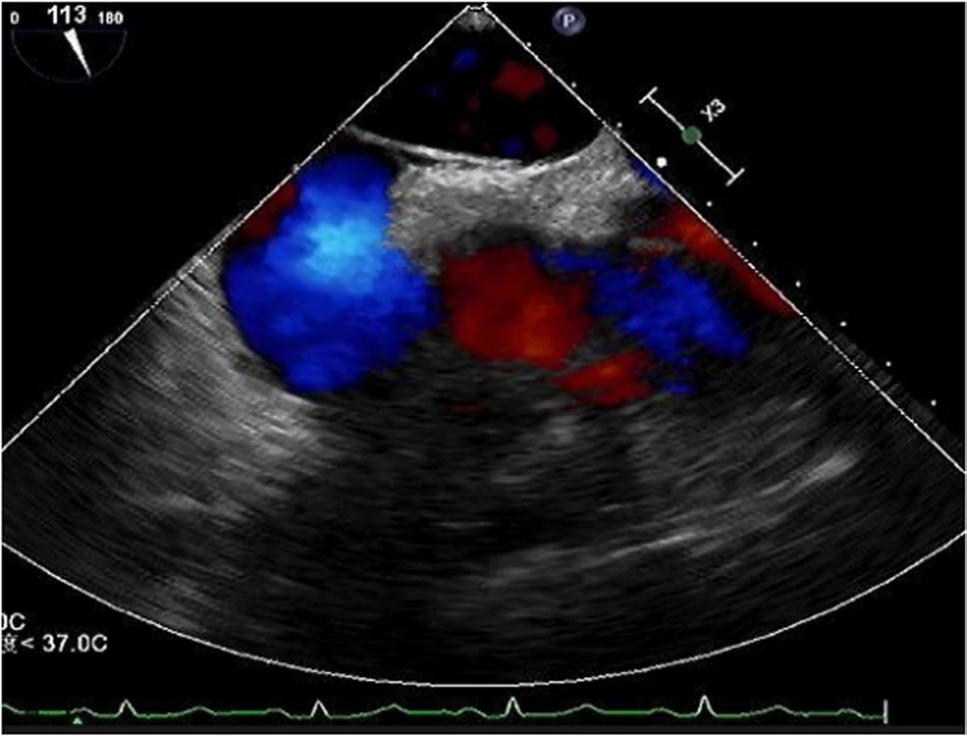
Transesophageal echocardiography (TEE) revealed a PFO
